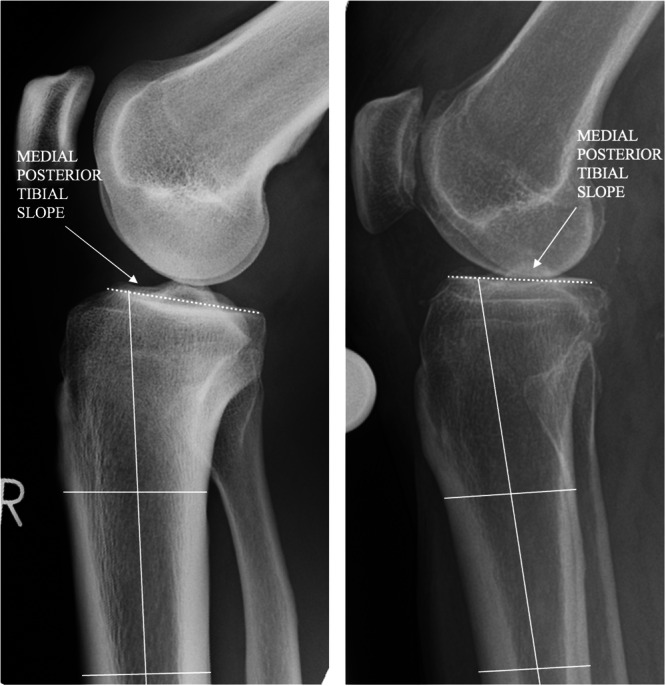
Measurement of MPTS. Lateral radiographs of a non‐OA knee (left) and a knee with medial compartment osteoarthritis (right) showcasing measurement of medial posterior tibial slope. MPTS, medial posterior tibial slope; OA, osteoarthritis.
